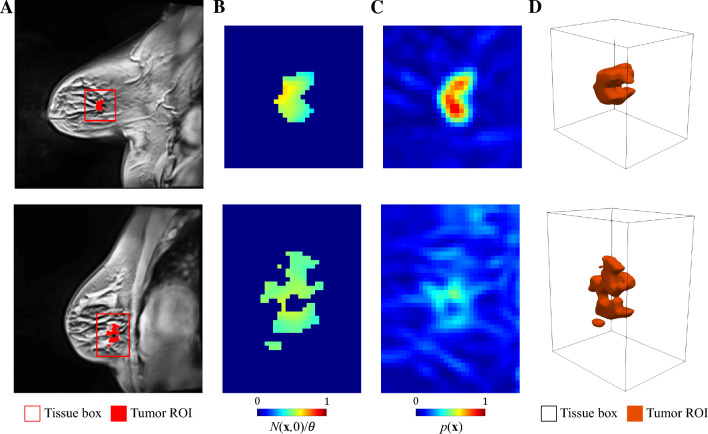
Patient-specific, MRI-informed tumor scenarios for the sensitivity analysis. Panels A-D show the in vivo MRI measurements characterizing the two TNBC cases considered in this study: a well-perfused tumor (top row) and a poorly perfused tumor (bottom row). Panel A presents a contrast-enhanced $$T_1$$-weighted sagittal image showing the patient’s breast anatomy and the tissue box containing the tumor region of interest (ROI) for each scenario. Panel B shows the corresponding tumor cell density maps over a sagittal section of the tissue box. These tumor cell density maps were calculated from ADC measurements obtained from DW-MRI data and normalized with respect to the tissue carrying capacity ($$N(\textbf{x},t)/\theta$$, see Sect. 2.3.1). Panel C depicts the local perfusion map over the same sagittal section of the tissue box for each tumor case. These perfusion maps were computed from DCE-MRI data ($$p(\textbf{x})$$, see Sect. 2.3.3). Finally, panel D shows a 3D rendering of each tumor ROI within the corresponding tissue box
